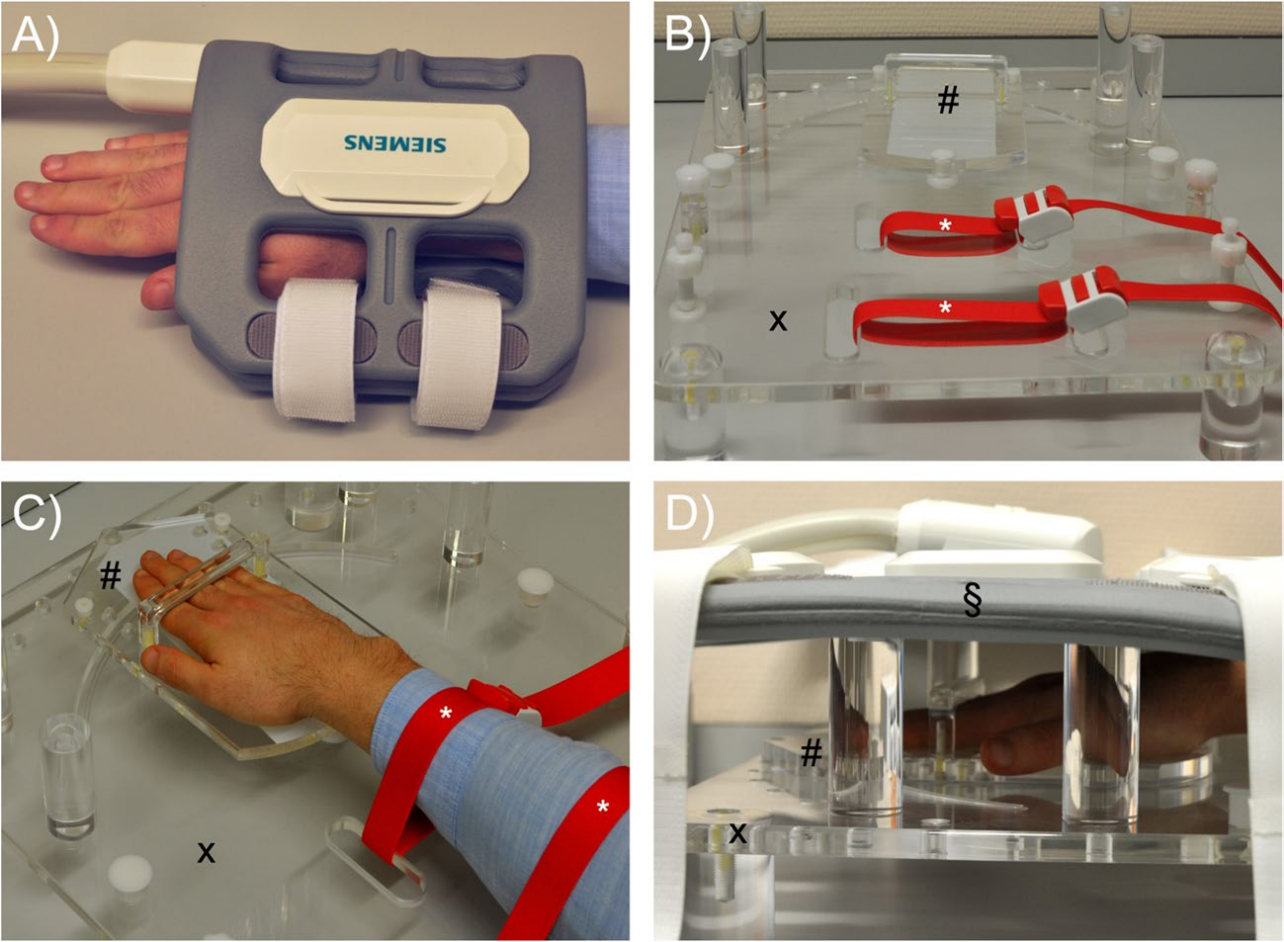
Details of the MR imaging setups. A) During static imaging, morphologic sequences were acquired with a standard 4-channel flex coil centered around the wrist. B-D) Setup for real-time MRI measurements: The MRI-compatible motion device is shown without (B) and with (C) a patient’s right forearm and hand. The hand was positioned on the mobile sliding plate (#) and, thus, guided along a pre-defined semi-circular range on the immobile base plate (x). Tourniquets were used to immobilize the forearm (*). The loaded and operational device (D) was covered with an 18-channel body coil (§) placed on top of the transparent spacers and centered around the wrist. A 32-channel spine matrix coil was positioned underneath the device – left out for clarity
